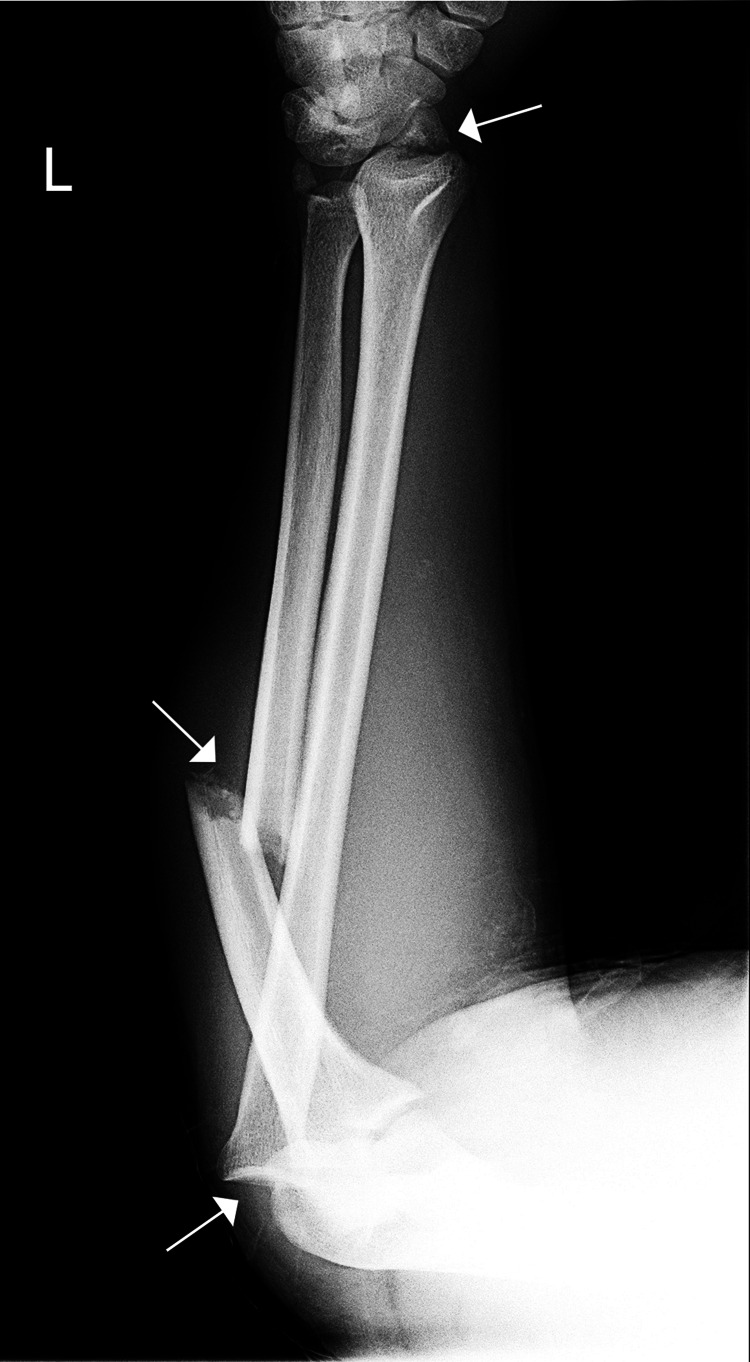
Initial X-rays of the forearm Proximal radioulnar dislocation (lateral radial head dislocation), an ulnar shaft fracture and radiocarpal dislocation.
